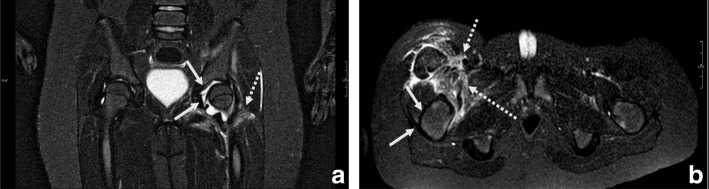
Most significant coxofemoral joint MRI findings performed on the third day after admission in both cases. (a). Case I: coxofemoral joint MRI (frontal projection) showed left coxofemoral intra-articular effusion (continuous arrows) with oedematous-flogistic muscular involvement (dotted arrow) but no bone involvement. (b). Case II: Coxofemoral joint MRI (axial projection) showed mild right hip joint effusion (continuous arrows) and fasciitis muscular effusion (dotted arrows); no bone lesions were detected
